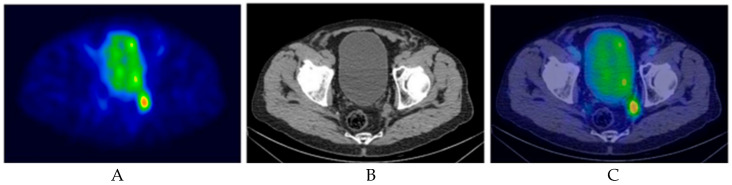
[99mTc]Tc-PSMA-I&S SPECT/CT. (A) Identification of a suspicious lymph node at the level of the left obturatory region, as shown in SPECT, (B) CT and (C) SPECT/CT—axial view (Reprinted with permission from Quarta et al. [66], 2024, Creative Commons CC BY).
